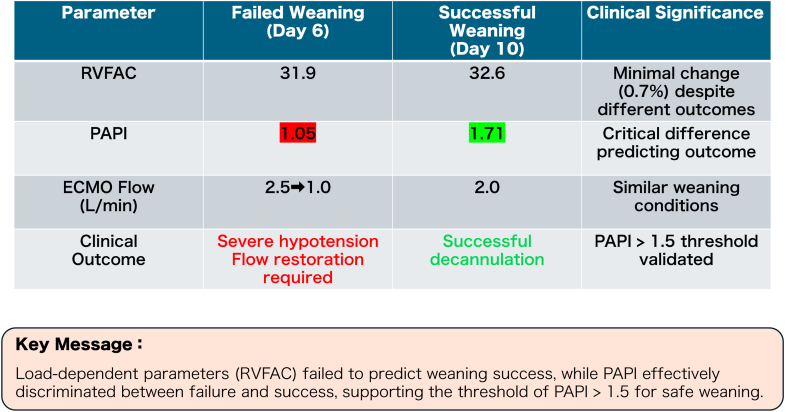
Comparison of Weaning Parameters in Case 1 Comparison of hemodynamic and echocardiographic parameters between failed weaning (day 6) and successful weaning (day 10) in case 1. Although right ventricular fractional area change (RVFAC), a load-dependent parameter, showed a minimal change (31.9% vs 32.6%), the pulmonary artery pulsatility index (PAPI) showed a significant improvement (1.05 vs 1.71), correctly predicting weaning success. This supports the utility of PAPI >1.5 as a weaning threshold. ECMO = extracorporeal membrane oxygenation.
